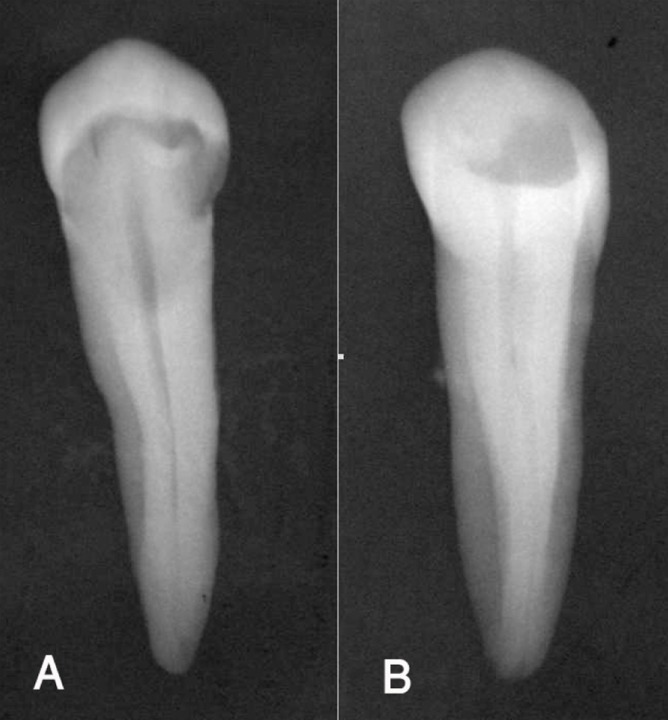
A: Straight, B: 20 degree angulated radiograph
